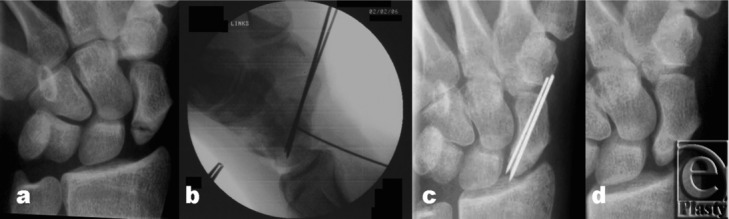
X-rays of a proximal located scaphoid nonunion. A 28-year-old male patient suffering from scaphoid nonunion for 2 years. (*a*) Preoperatively. (*b*) Intraoperatively with a small k-wire for fixation of the bone graft, palmar approach. (*c*) 6 weeks postoperatively. (*d*) 12 weeks postoperatively.
